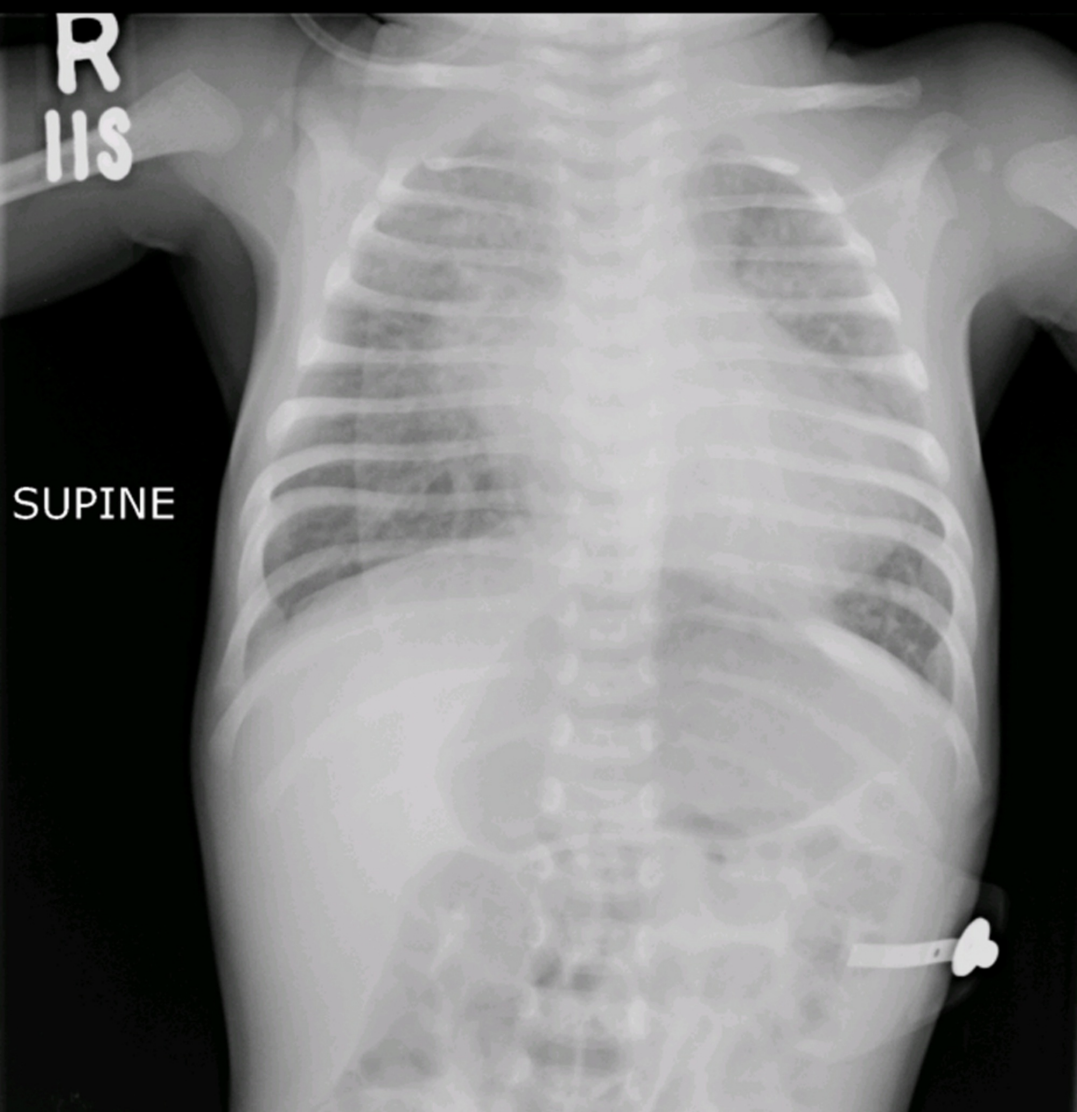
Chest X-ray Rounding and elevation of the cardiac apex along with pulmonary vascular congestion, suggestive of congenital heart disease with pulmonary plethora.
